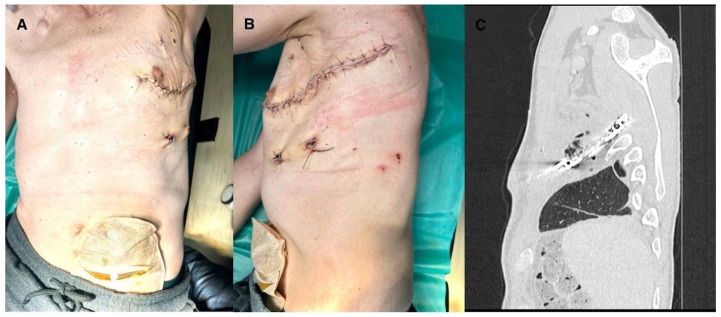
(A). Patient after surgery anterior view. (B). Patient after surgery lateral view. (C). Patient after surgery CT scan lateral view (This figure should be printed in colour).
